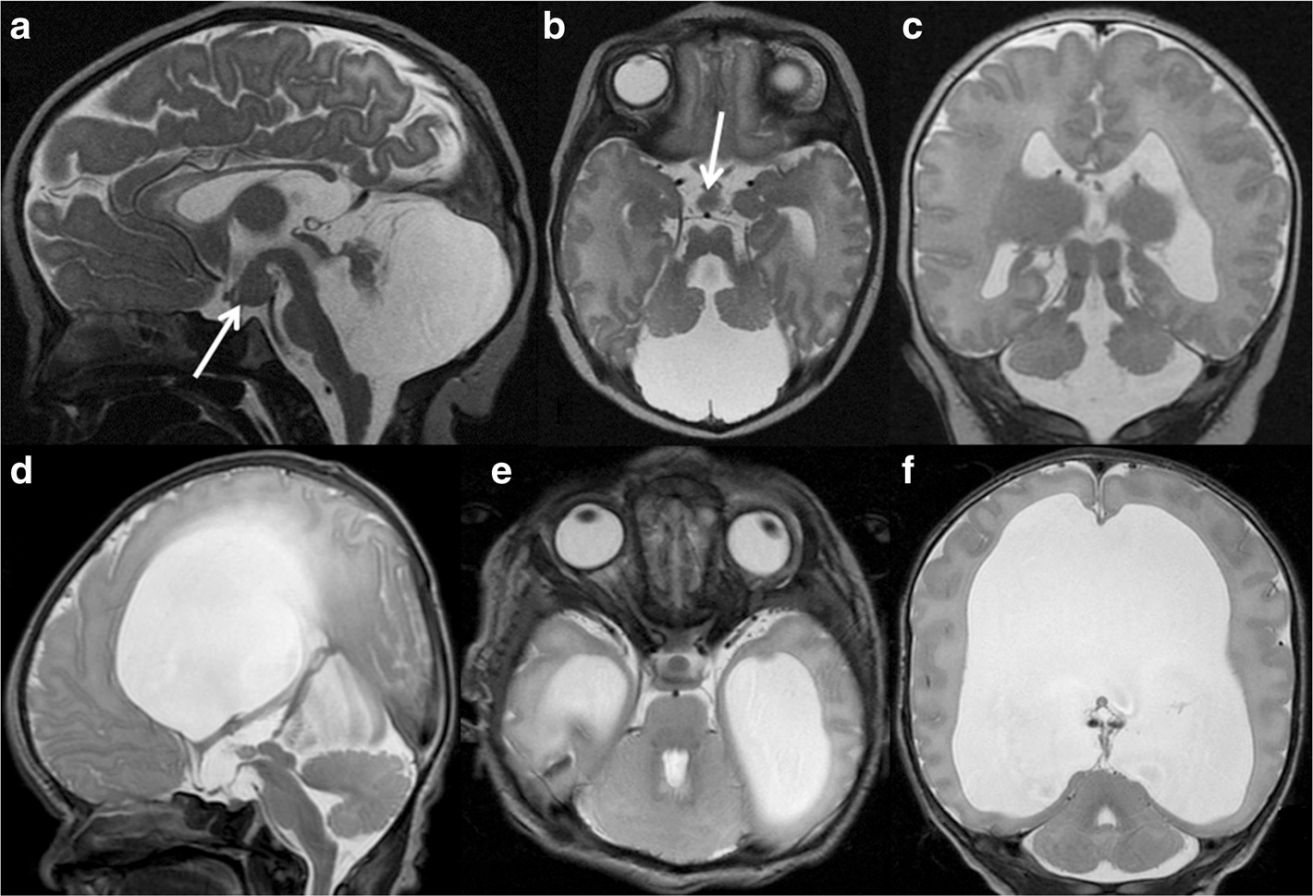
a, Sagittal, b, Axial, and c, Coronal T2-weighted MR images of a 2-day-old term male newborn with Oral-Facial-Digital syndrome type VI (a phenotype of Joubert syndrome) show severe hypoplasia of the cerebellar vermis and both cerebellar hemispheres, the characteristic molar tooth sign including thickened and elongated superior cerebellar peduncles and a deepened interpeduncular fossa, and a hypothalamic hamartoma (arrows)(reprinted with permission from Poretti A et al., AJNR Am J Neuroradiol, 2008;29:1090–91). d, Sagittal, e, Axial, and f, Coronal T2-weighted MR images of a 5-day-old term male neonate with rhombencephalosynapsis reveal continuity of the cerebellar hemispheres, dentate nuclei, and superior cerebellar peduncles without a midline intervening vermis. On the sagittal image, no primary fissure is seen. In addition, dysplasia of the tectal plate, obstruction of the Sylvian aqueduct at the level of the inferior colliculi, and marked supratentorial hydrocephalus. Note that the dentate nucleus is visible on a midsagittal image, while in normal anatomy the vermis separates the dentate nuclei in the midline
